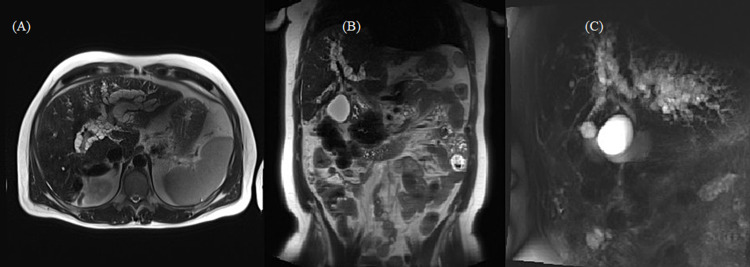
(A and B) MRI with (C) MRCP showed multiple grape-like cystic structures surrounding the intrahepatic portal vein branches MRCP: magnetic resonance cholangiopancreatography
